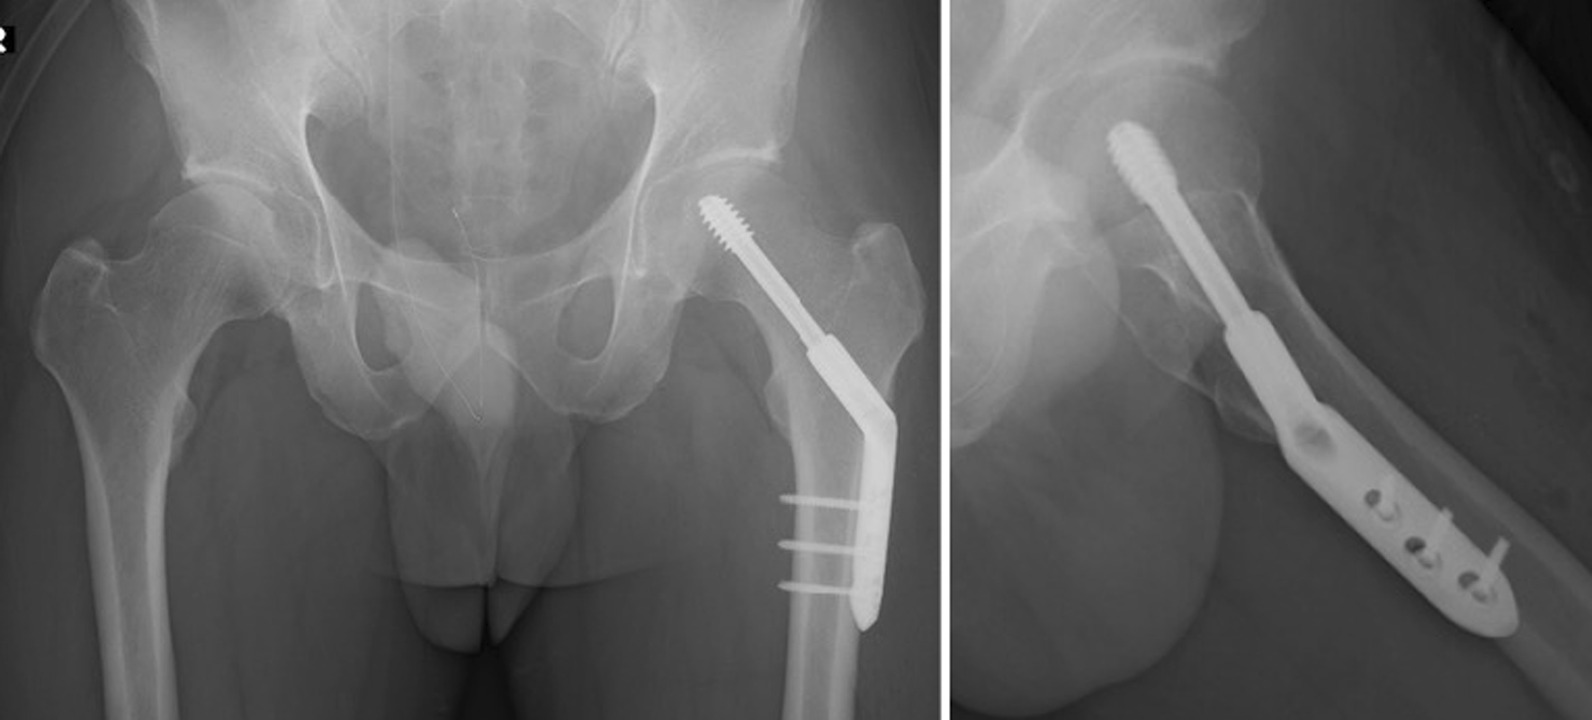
Postoperative images. Anteroposterior view (left) and Lauenstein view (right) of hip joint plain radiography showing that the compression hip screw was correctly inserted
